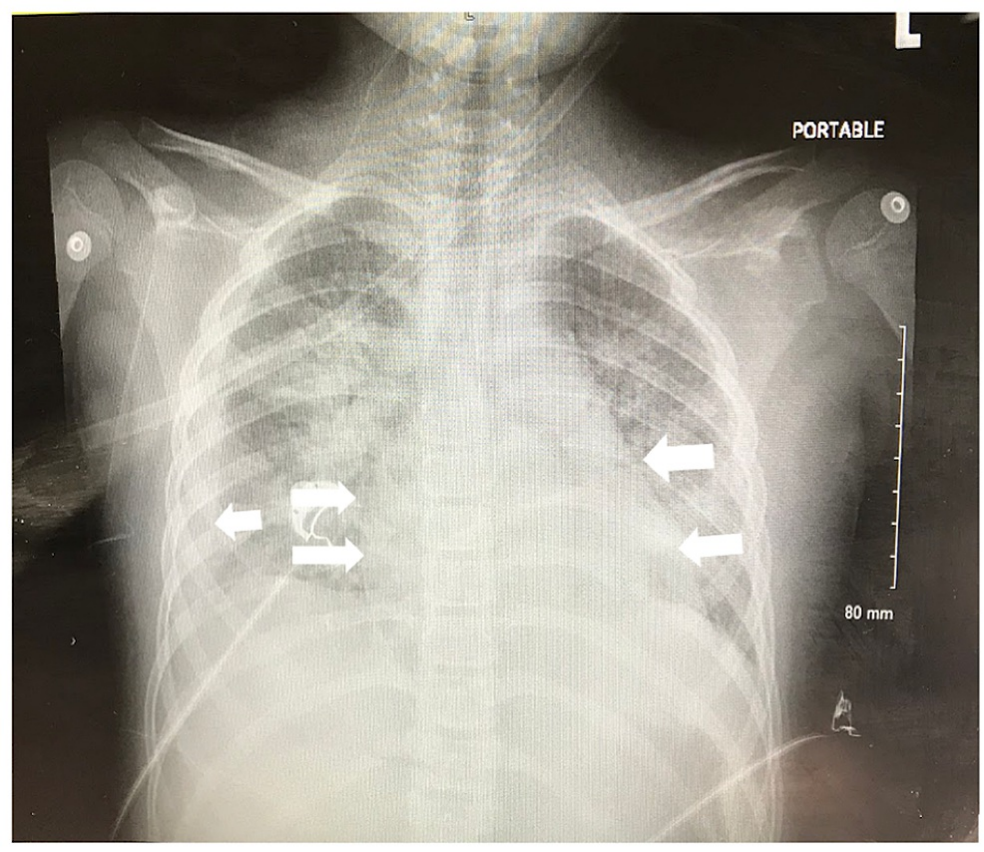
Repeat anterior posterior (AP) chest x-ray showing right-sided pleural effusion with cardiomegaly and extensive bilateral infiltrates.
